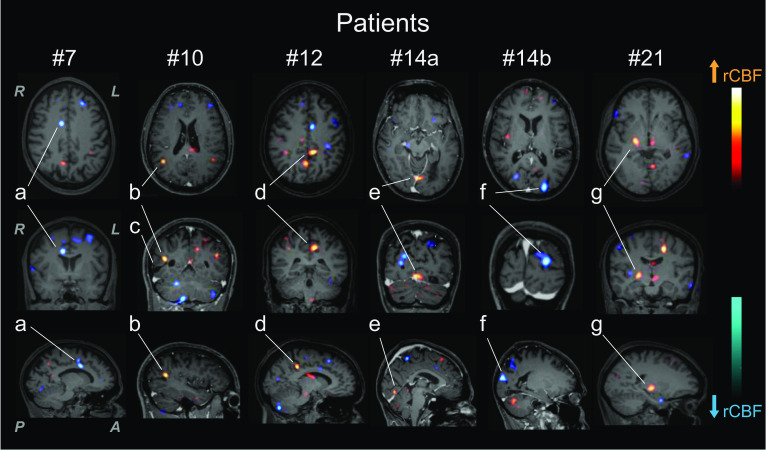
Subtraction SPECT coregistered to MRI (SISCOM). *Patient #7* presented decreased rCBF in the anterior cingulate cortex (a), associated with recovered anxiety, addiction and psychosis after pulse therapy. *Patient #10* presented increased rCBF in the right inferior parietal lobe (b) adjacent to a vasculitic insult (c), possibly due to luxury perfusion of the neighbouring cortex after treatment, and associated with the recovery of memory and hallucinations. *Patient #12* presented increased rCBF in the precuneus, posterior cingulate cortex (d), and hypothalamus, associated with post-treatment persistent psychosis, aggressivity, and behaviour modification. *Patient #14* presented two functional status after a left posterior cortex stroke: increased rCBF (e) (#14a) in the left medial occipital lobe (reperfusion) possibly related to post-treatment recovery from the hallucinations, and decreased rCBF (f) (#14b) in the posterior occipital lobe (worsening of ischemia). *Patient #21* presented increased rCBF overlapping with the infarcted right basal ganglia (g), possibly representing luxury perfusion, and associated with the improvement in depressive symptoms
